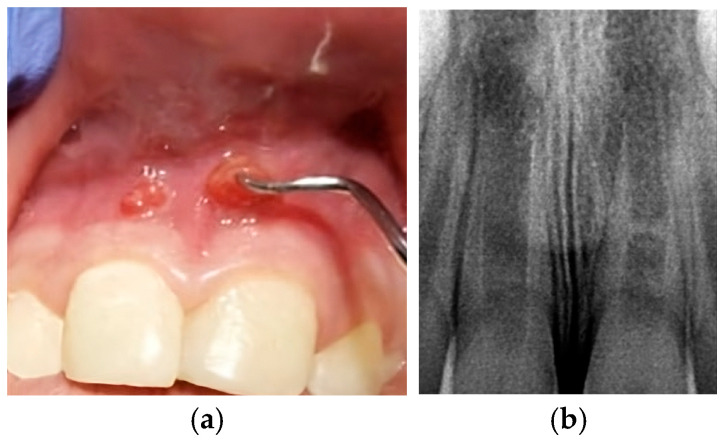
Clinical Case 2: (a) Intraoral photograph taken during the initial visit. (b) Periapical radiograph taken during the initial visit.
